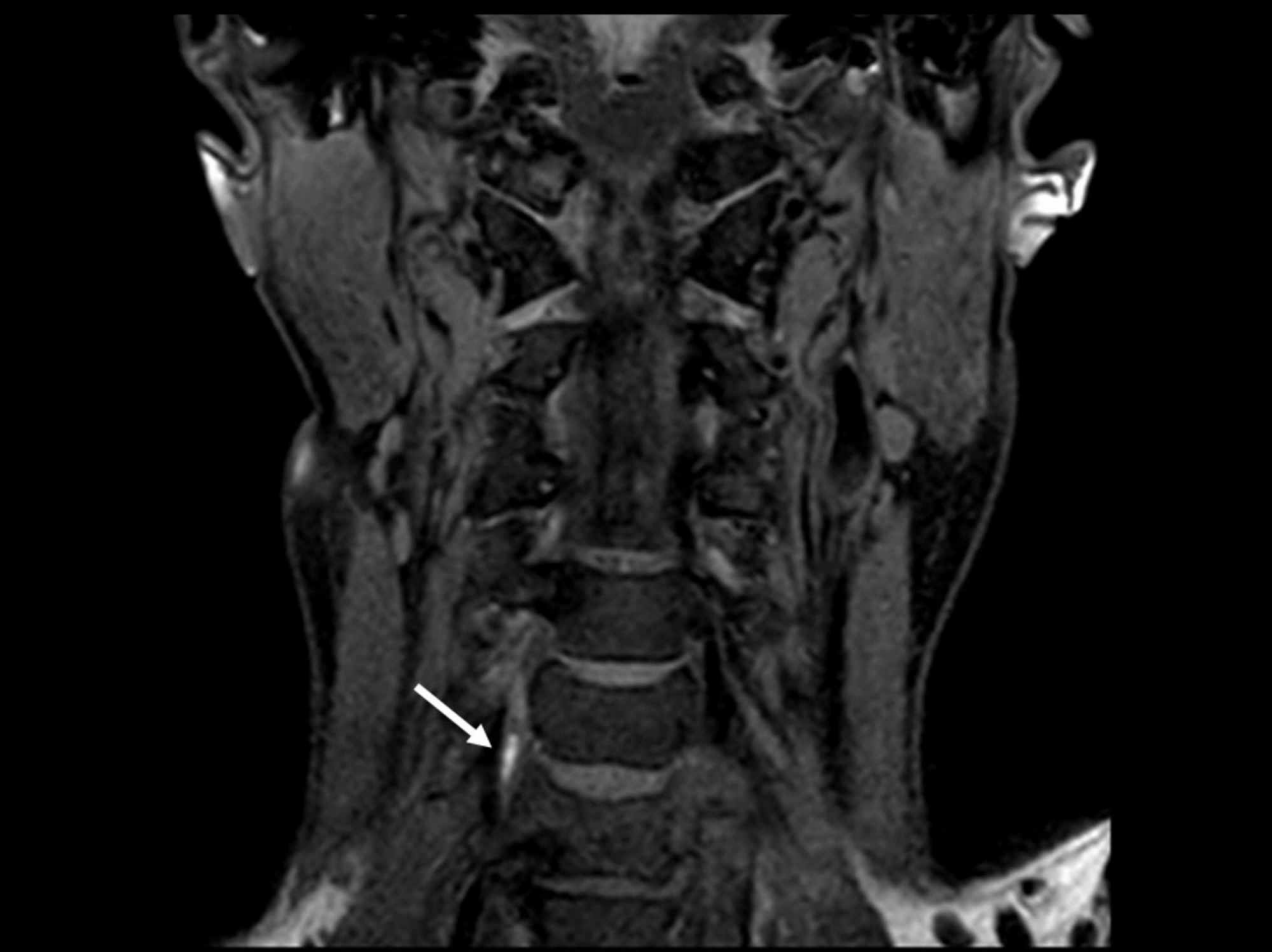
A coronal cervical T1-weighted image. The image shows a high-signal-intensity lesion (arrow) at the right vertebral artery, suggesting traumatic dissection.
